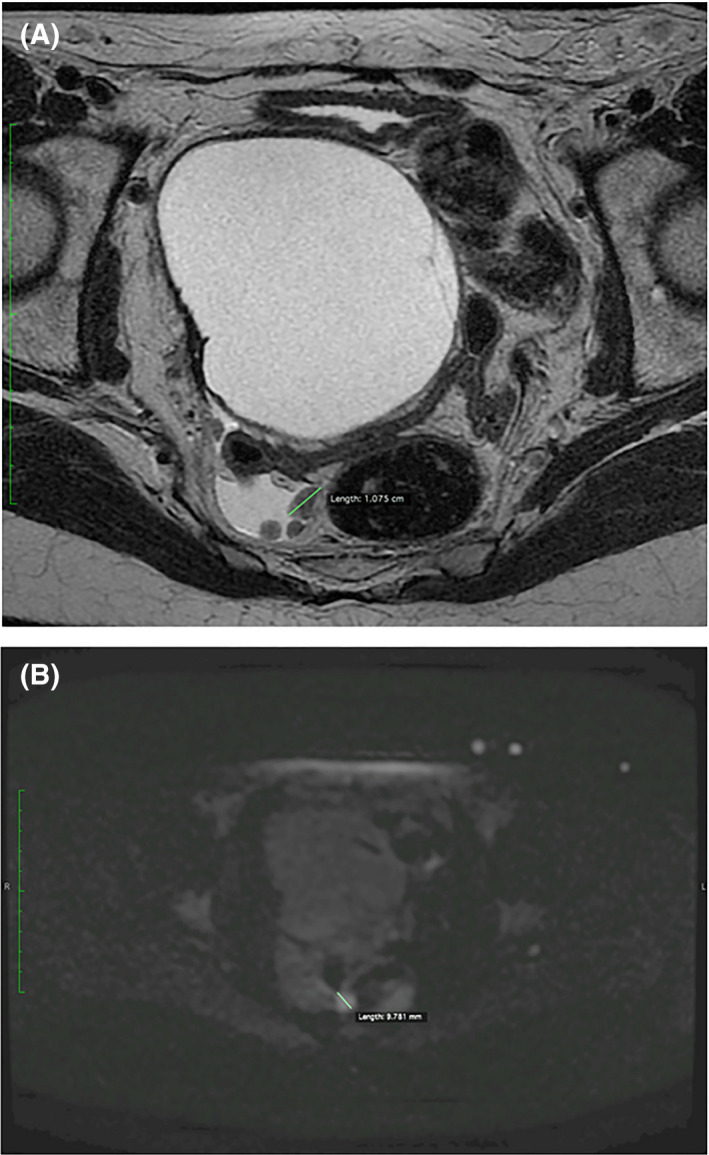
Nodes in the pelvis with restricted diffusion, largest node is 1.075 cm in diameter and ascites (magnetic resonance imaging scan performed on 22 December 2016)
